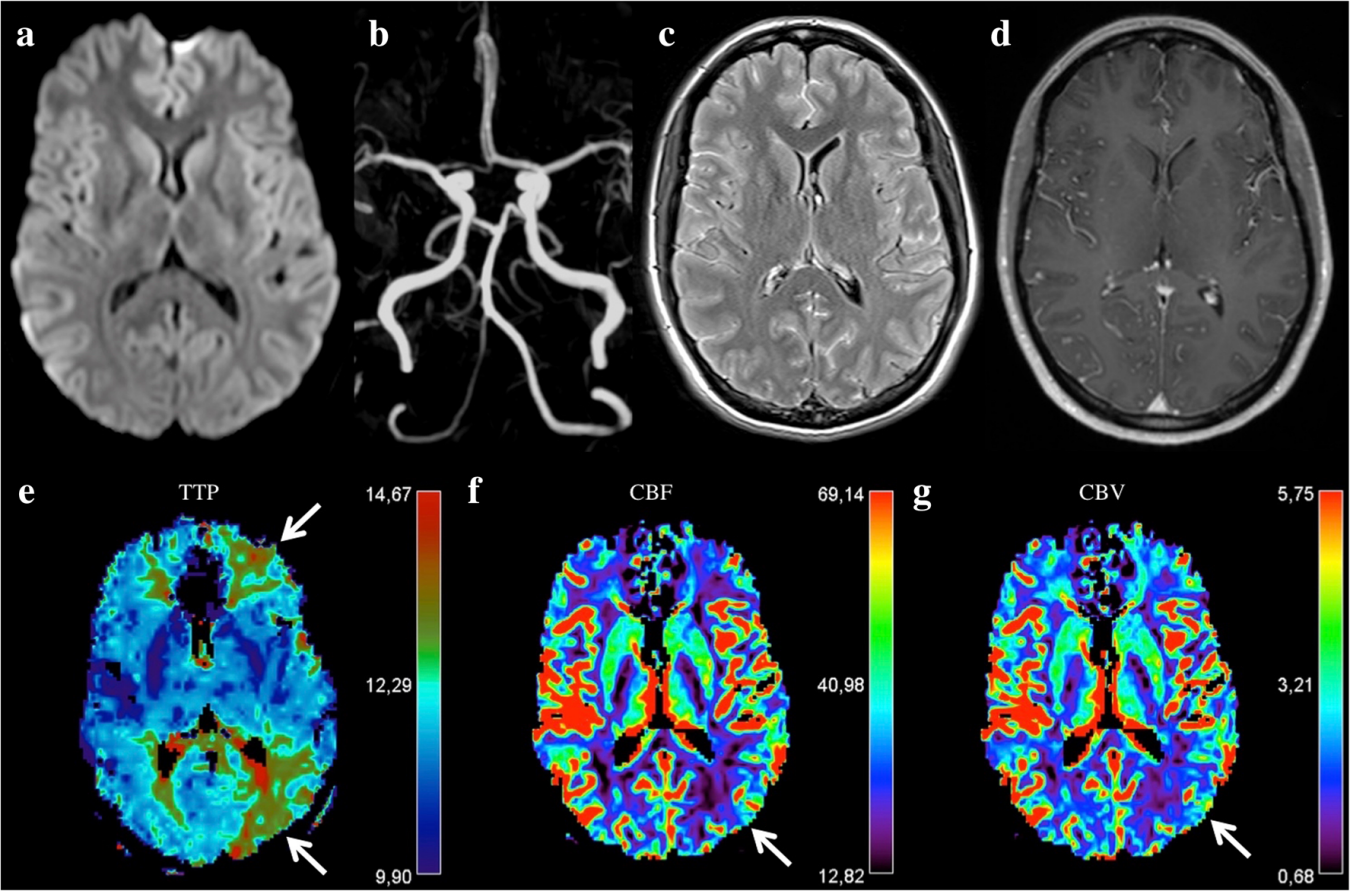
A 61-year-old woman with headaches with associated neurological deficits and lymphocytosis (HaNDL) presenting with motor and language deficits. No altered DWI (a), no arterial occlusion (b). Hyperintensities on FLAIR (c) and enhancement (d) in the cortical sulci may be seen related to lymphocytic meningitis. Hypoperfusion can be seen in more than one arterial territory (e–g, arrows)
